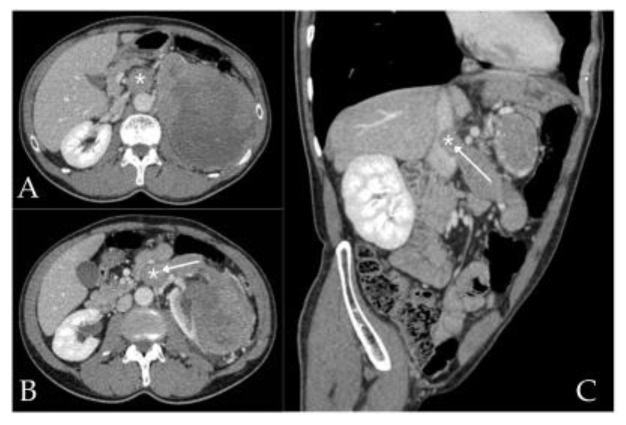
Contrast-enhanced computed tomography of locally advanced left-sided RCC (cT3bN0M0) with venous involvement (Level I)—multiple venous/nephrographic phase sections: (A) Axial (upper lumbar): large upper pole primary tumor (13/12 cm), with extensive intratumoral necrosis, and a significantly dilated left renal vein, occupied by the tumor thrombus (white asterisk); (B) Axial (lower lumbar): evident vascular invasion, with a displaced and markedly thicker left renal vein, molded around an extensive tumor thrombus (white asterisk), which is expanding towards the IVC from the left renal hilum (direction of white arrow); (C) Oblique (sagittal rotation in multi-planar reconstruction): expansion of tumor thrombus throughout the renal vein (direction of white arrow), reaching its peak cephalad extent (white asterisk) into the IVC lumen (≤2 cm above the ostium of the left renal vein).
